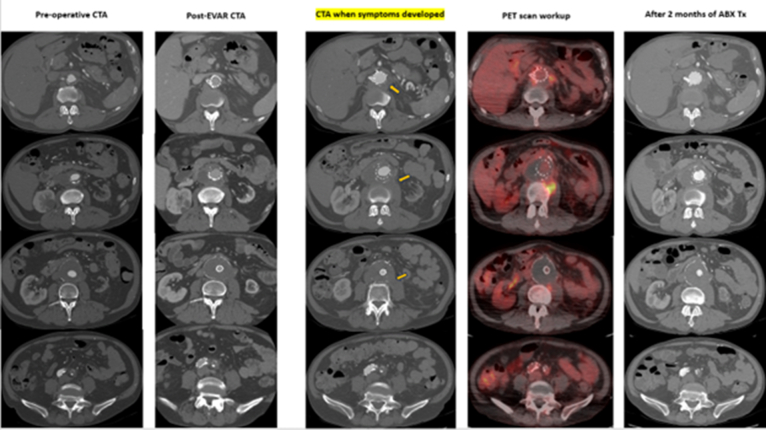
Captured timeline of the delayed development of *Bartonella henselae* endograft infection after endovascular aortic repair (EVAR). Arrows show enlarged retroperitoneal lymph nodes, which were confluencing, covering the abdominal aortic aneurysm (AAA) sac and neck near circumferentially. Positron emission tomography (PET) scan was positive. Antibiotic therapy (*ABX Tx*) was ineffective. *CTA*, Computed tomography angiography; *WBC*, white blood cell.
